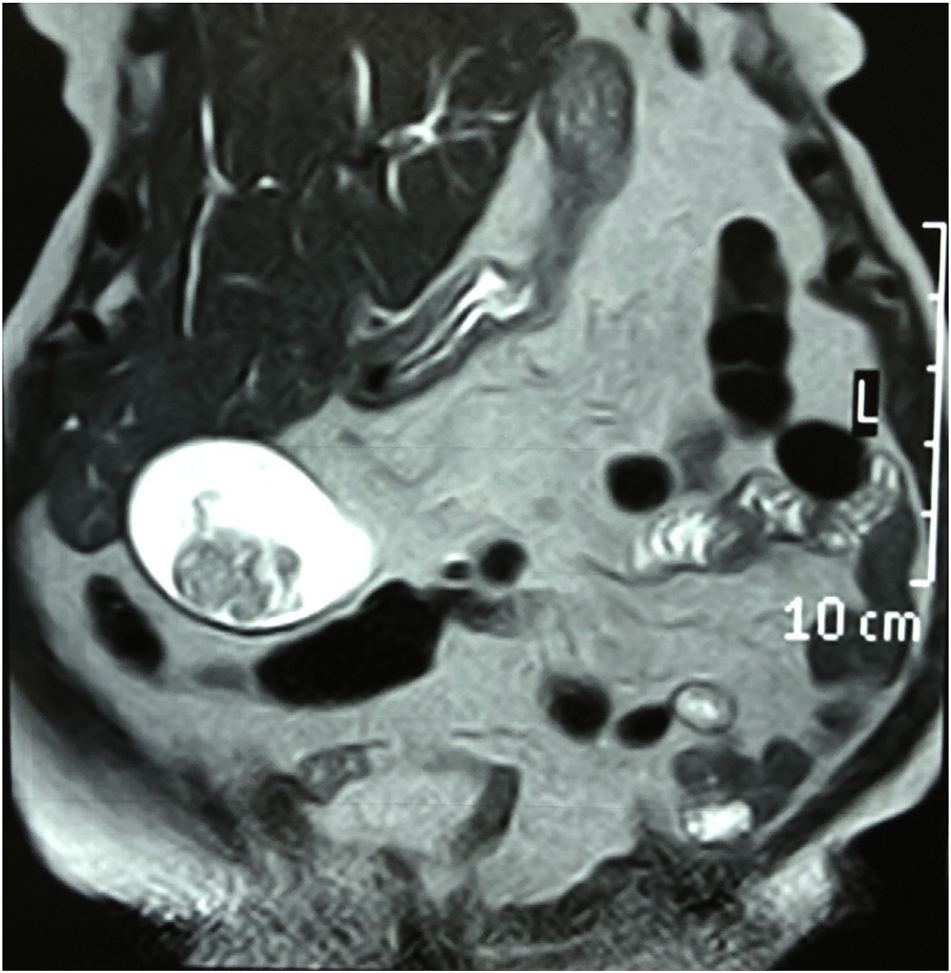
A T-2 weighted MRI picture showing a poly inside the lumen of the gall bladder.
